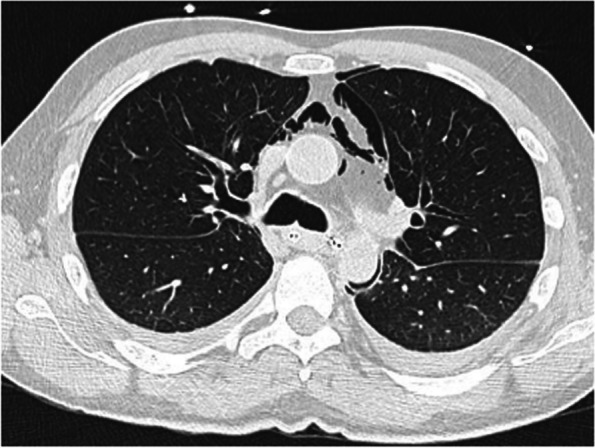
CT scan with contrast revealed a pneumomediastinum, right apical pneumothorax, bilateral pleural effusion, and cervical emphysema
